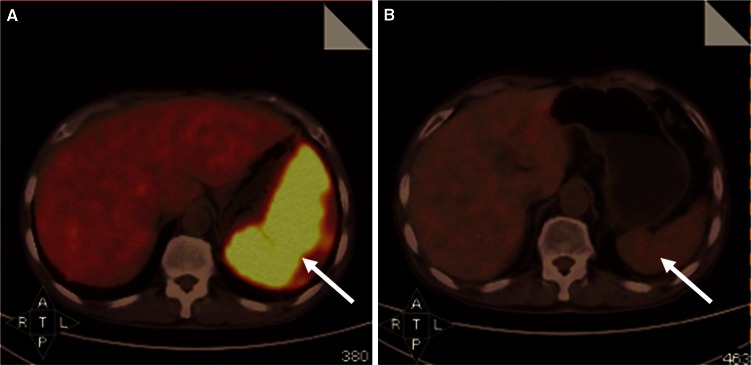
Comparison of PET–CT images of patient no. 2 who was highly suspected as lymphoma-associated HLH by PET–CT before lymphoma-chemotherapy and after treatment. a Initial PET–CT scan revealed FDG-avid splenomegaly with a SUV_Sp_ of 10.5 but no disease elsewhere (*arrow*). b Follow-up PET–CT scan was performed after receiving two cycles of EPOCH chemotherapy. It revealed significantly reduced spleen with no FDG-hypermetabolism (*arrow*). Until the date of cutoff time, the patient has survived for 652 days
